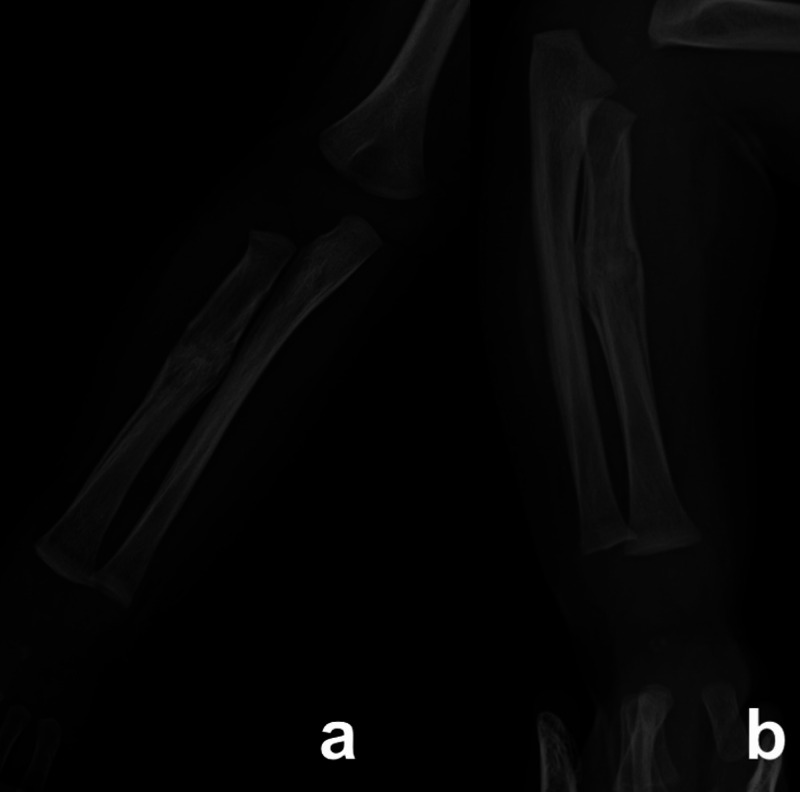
Radiographs at four weeks of treatment Radiographs show a well-united radius fracture in the posteroanterior (a) and oblique views (b)
